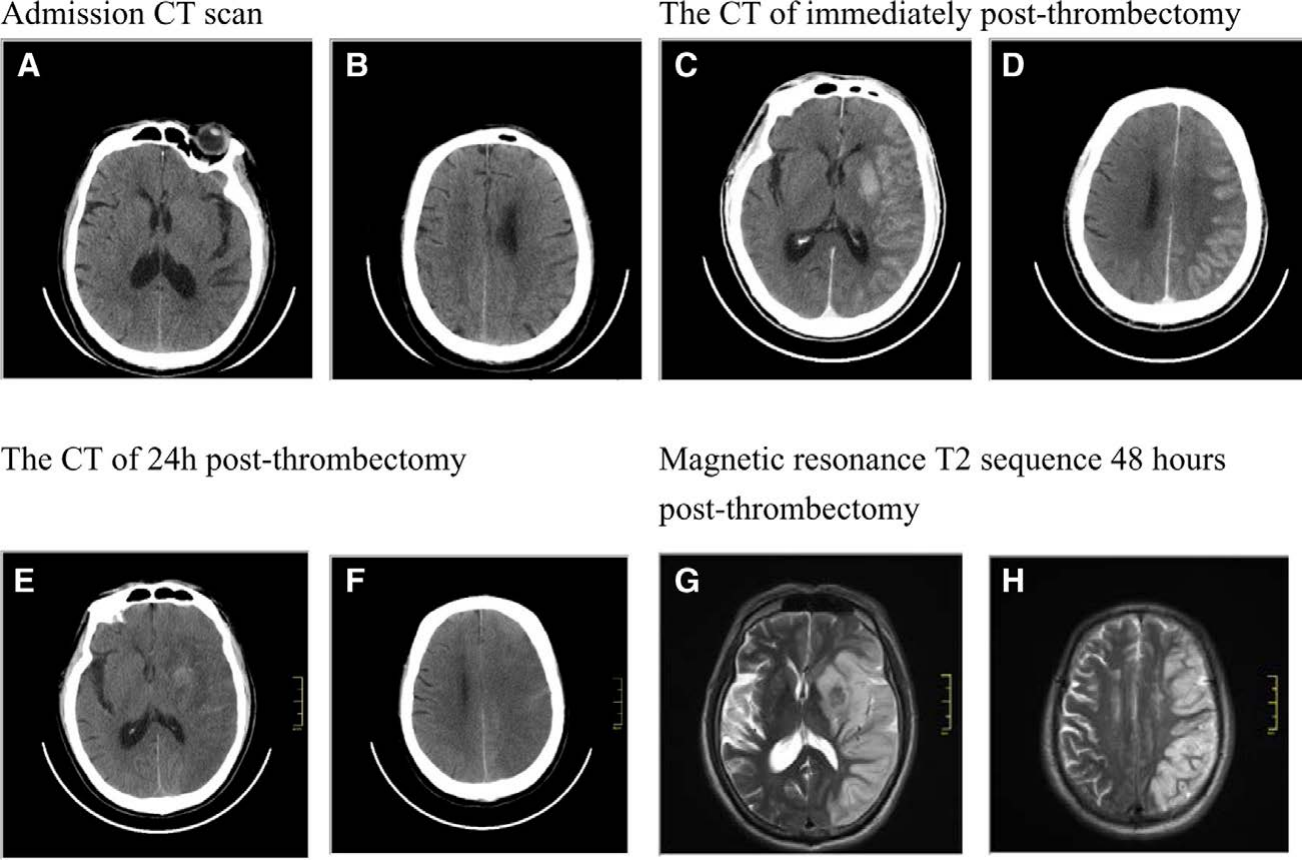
Example of a positive hyperdense lesion patient. The patient with baseline ASPECTS of 7, post-ASPECTS was 2 (caudate nucleus and posterior limb of the internal capsule were exempt), and mRS was 5 on 90 days followed-up. (A and B) Admission CT scan; (C and D) CT of immediately post-thrombectomy; (D and E) CT of 24 h post-thrombectomy; (F and G) magnetic resonance T2 sequence 48 hours post-thrombectomy. ASPECTS = Alberta Stroke Program Early CT Score, mRS = modified Rankin Scale.
